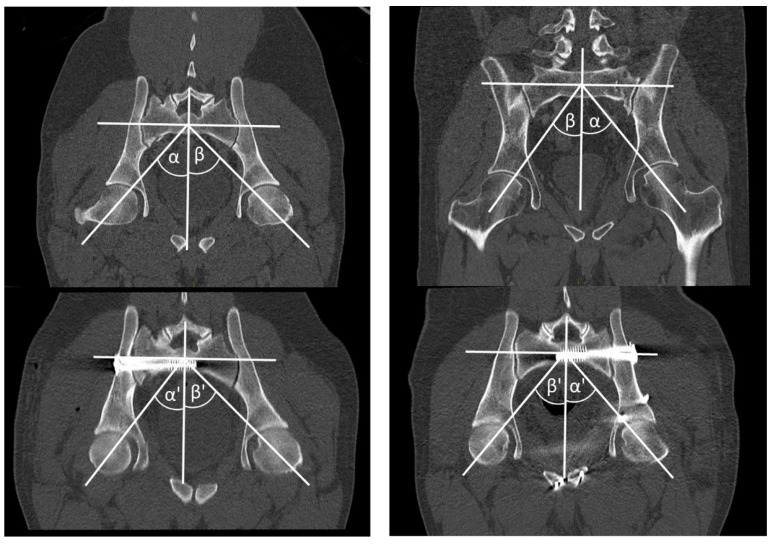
Measurement of the mechanical load-bearing axis in MPR 2. ILBA (α) and CLBA (β) in preoperative CT scan and ILBA (α’) and CLBA (β’) in postoperative CT scan.
